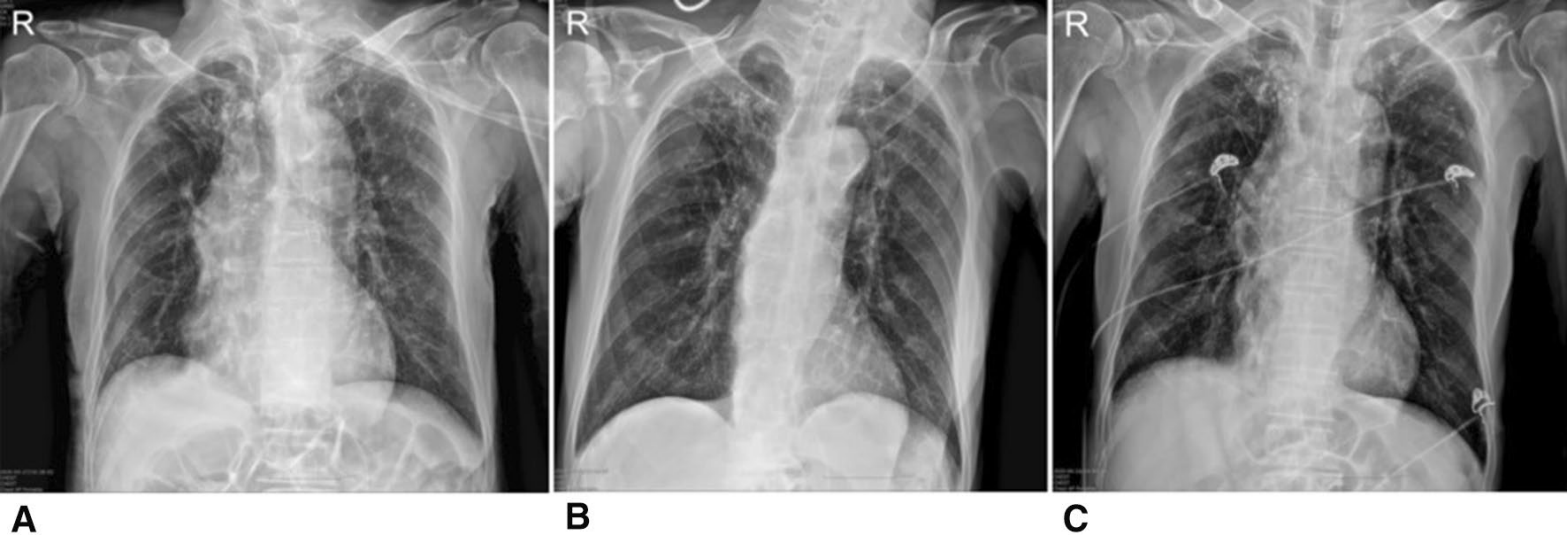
Serial chest radiographies of patient C. A Shows post-inflammatory fibrosis and nodules, at both upper lobes, GGO at both lower lung field (Esp. LLL) at initial chest radiograph. B Taken 5 days after shows no interval change of pneumonia at both lower lungs. C Taken 3 weeks after shows slightly improvement of pneumonia
